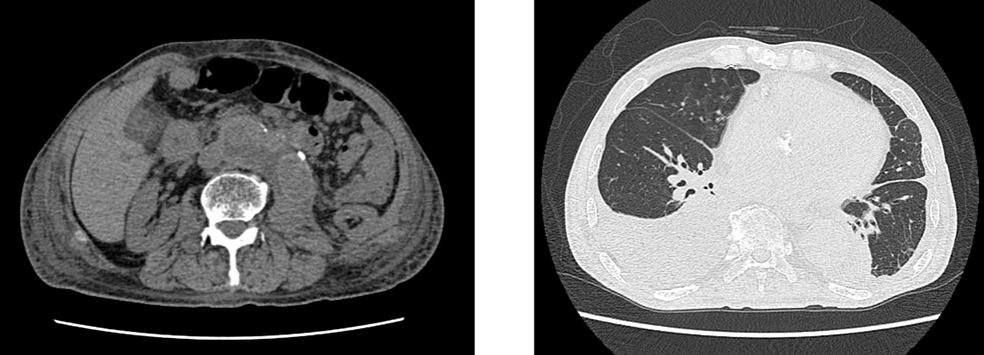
Computed tomography findings The left kidney was atrophied compared with the right kidney. Bilateral pleural effusion and right atelectasis are observed. There is no interstitial lung disease or alveolar hemorrhage. An abscess was suspected in the structure on the right side of the artificial blood vessel.
